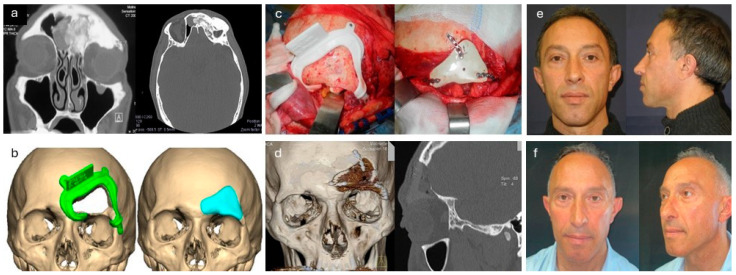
Resection and primary reconstruction of a fronto-orbital osteoma. (a) Preoperative CT scan revealing a heterogeneous fronto-orbital expansive process extending to the roof of the orbit and frontal sinus. (b) Customized cutting guide implant digital planning. (c) Intraoperative placement of the cutting guide for executing the osteotomy and subsequent fixation of the implant. (d) Postoperative CT scan. (e) Aesthetic outcome at the 3-month follow-up. (f) Aesthetic outcome at the 24-month follow-up.
